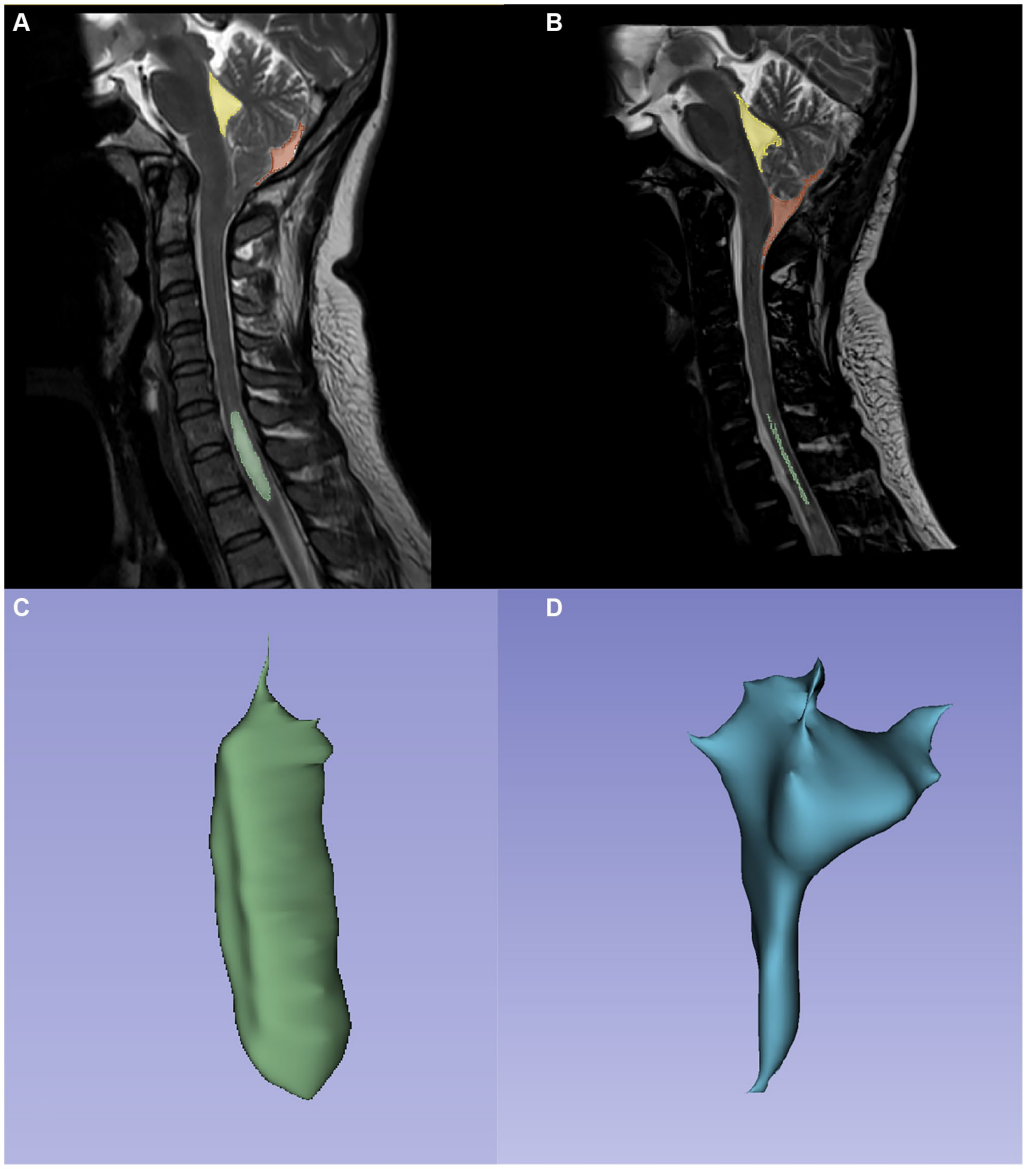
Panels (A,B) are the preoperation and postoperation MRI images of a 60-year-old male patient who underwent PFDRT treatment. Both in (A,B), yellow, fraction and green represent the fourth ventricle, cistern magna, and SM area, respectively. Panels (C,D) are the SM and cisterna magna models drawn by 3Dslicer, respectively.
